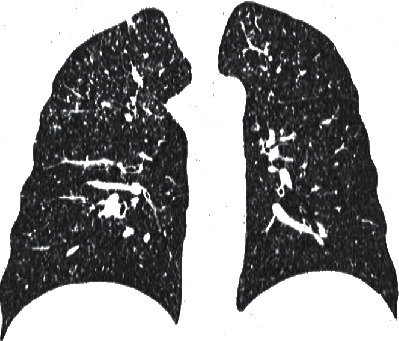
Coronal plane chest CT with the presence of innumerable small (2–4 mm) pulmonary nodules with a centrilobular predilection, consistent with miliary tuberculosis.
